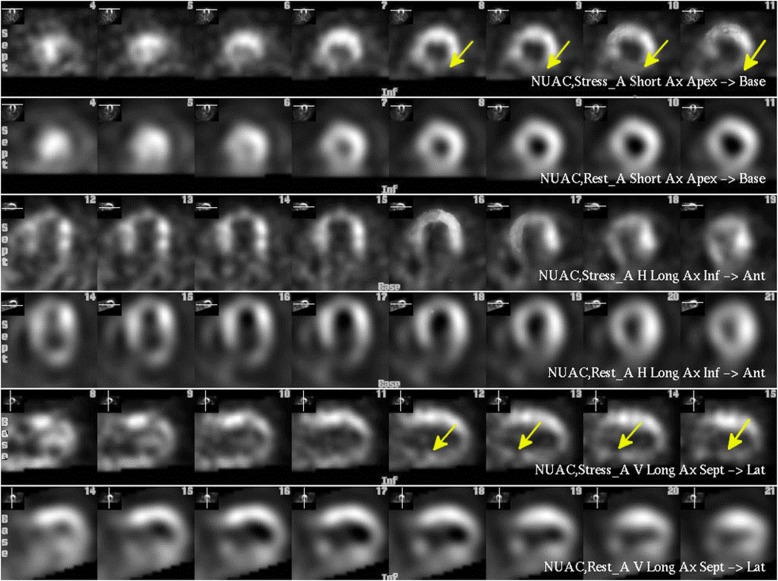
Myocardial perfusion SPECT scintigraphy demonstrating the short axis (SA) slices (top), vertical long axis (VLA) slices (middle), and horizontal long axis (HLA) slices (bottom). The top row of each pair is at stress and the bottom at rest. The yellow arrows show the perfusion defect in the inferior wall under physiological stress
